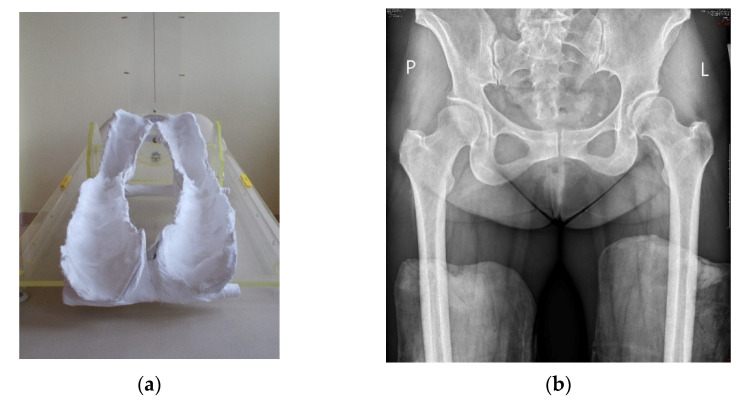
Plaster molds with 20-degree internal rotation of leg (a) in which AP digital radiographs were taken (b).
